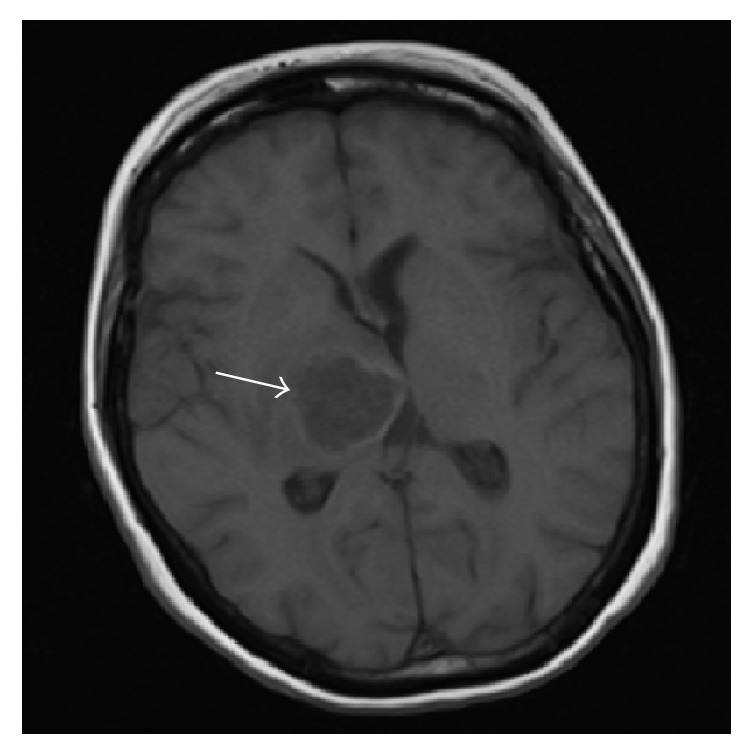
MRI brain with contrast showing intra-axial centered abscess (arrow) in the right thalamic region with associated right to left midline shift and downward herniation.
